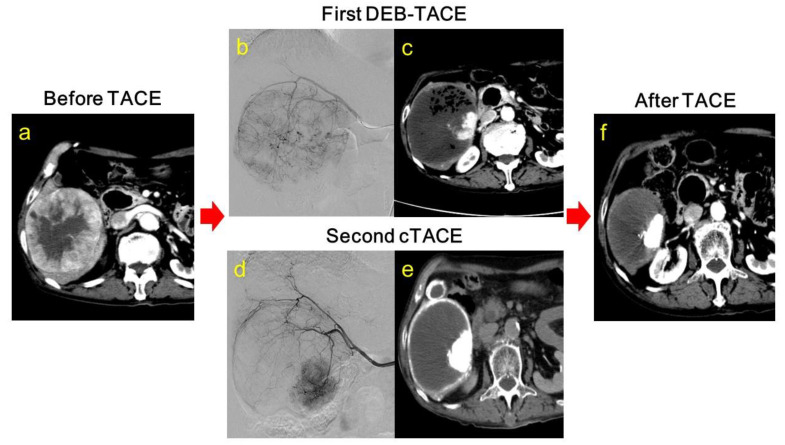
Radiographic images showing progress of treatment in 54-year-old female study participant with hepatocellular carcinoma: (a) dynamic computer tomography image from early treatment phase before transcatheter arterial chemoembolization (TACE) procedures; (b) angiographic image taken during the first drug-eluting beads-transcatheter chemoembolization (DEB-TACE) procedure; (c) dynamic computer tomography image from the early treatment phase a month after the first DEB-TACE procedure; (d) angiographic image taken during the second conventional TACE (cTACE) procedure; (e) regular computer tomography image taken immediately after the second cTACE procedure; and (f) dynamic computer tomography image taken a month after the second cTACE procedure.
